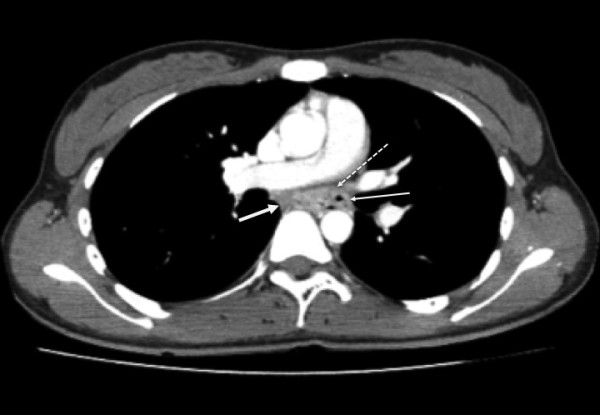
Chest computed tomography showed a mediastinal mass lesion at caudal side of tracheal carina approximately 3 cm diameter. The lesion contained partially air density part (thin solid line) and solid portion (bold solid line). Dotted line showed esophageal lumen.
